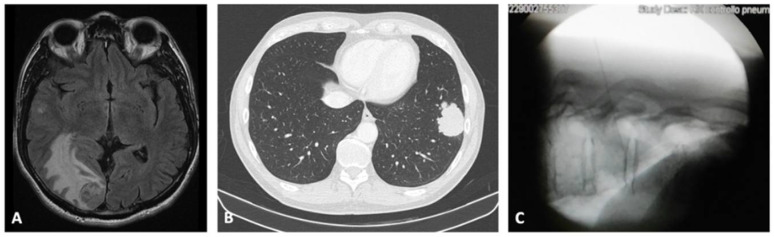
Case 2. (A) Axial T2WI/FLAIR MRI of the major brain lesion in the right occipital lobe. (B) axial CT scan of the rounded lung mass of the left lower lobe. (C) transthoracic needle aspiration with fluoroscopy guidance. MRI, magnetic resonance imaging; CT, computerized tomography.
